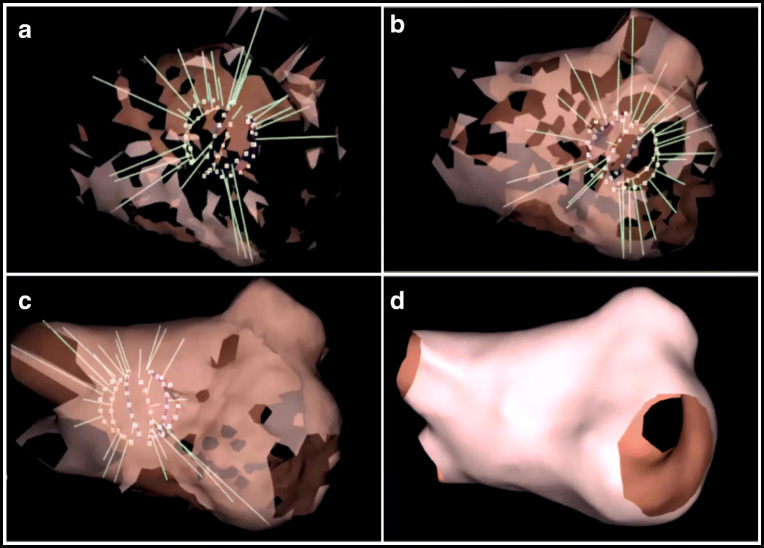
Anatomy reconstruction panels (a), (b), and (c) represent the build out of the LA anatomy with the ultrasound enabled AcQMap catheter. The green vectors on the map are originating from the 48 ultrasound transducers on the AcQMap catheter. The vectors are proportional to the distances travelled from the transducers towards the endocardial surface. Panel (d) represents the completed LA anatomy which will serve to project upon the electrical activation of the LA
